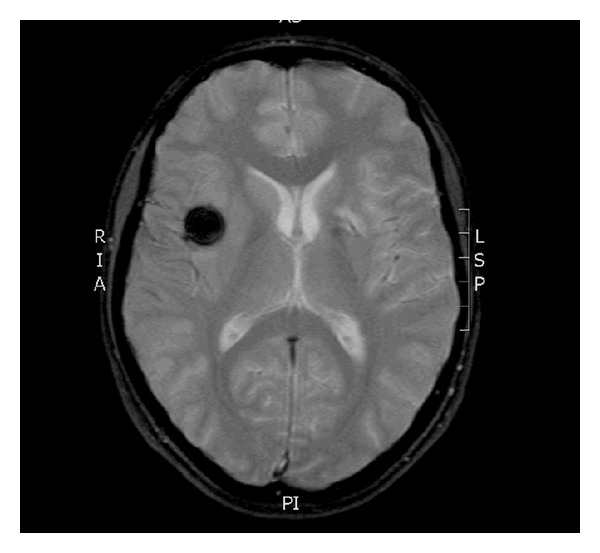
MRI-GE image scan 24 hours after Onyx embolization showing an area of low intensity at the site of embolic material within the aneurysm without ischemic complication.
